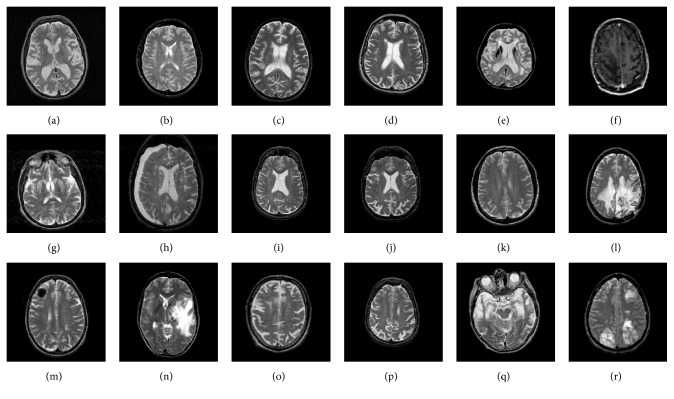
Brain MR images: (a) healthy brain; (b) AIDS dementia; (c) Alzheimer's disease plus visual agnosia; (d) Alzheimer's disease; (e) cerebral calcinosis; (f) cerebral toxoplasmosis; (g) Creutzfeldt-Jakob disease; (h) glioma, (i) herpes encephalitis; (j) Huntington's disease; (k) Lyme encephalopathy; (l) meningioma; (m) metastatic adenocarcinoma; (n) metastatic bronchogenic carcinoma; (o) motor neuron disease; (p) multiple sclerosis; (q) Pick's disease; and (r) sarcoma.
